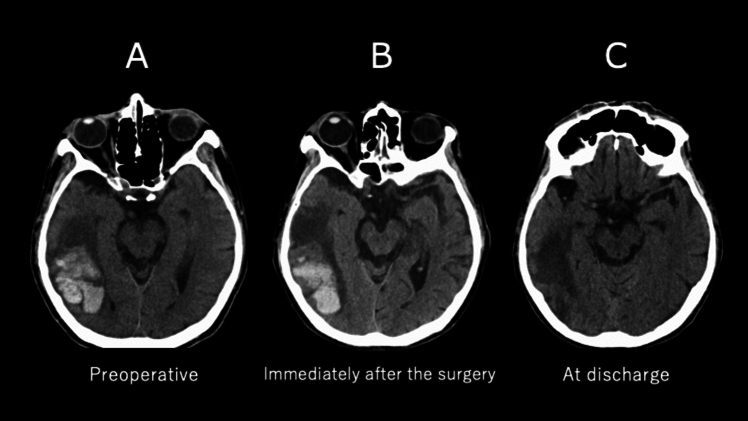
Computed tomographic images of a case with a large hemorrhagic lesion. A 62-year-old male had a hemorrhagic infarction in his right temporal lobe. He underwent a mitral valve replacement 6 days after the onset of the hemorrhagic infarction, and no enlargement of the hemorrhagic lesion was observed. A Preoperative image. B An image immediately after surgery. C An image at discharge
